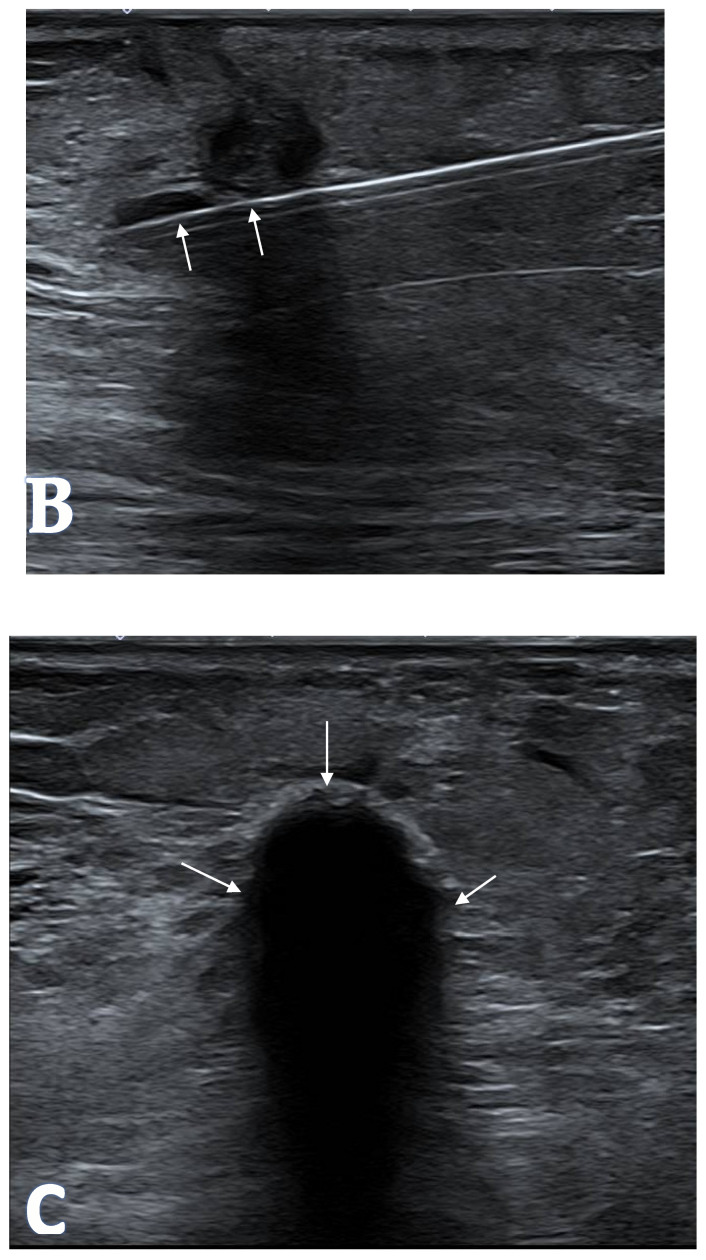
Ultrasound characteristics of a patient’s deltoid parathyroid autoimplant (male, 68 years old, on hemodialysis for 10 years) before and after cryotherapy (CA). (A) A 3.5 × 2.5 cm hypoechoic parathyroid autoimplant in the deltoid muscle before cryotherapy: normal parathyroid autoimplant on the left (white arrow) and hyperplastic parathyroid autoimplant on the right (bolded white arrow). (B) Cryo probe (white arrow) was inserted in the hypoechoic parathyroid autoimplant. (C) Hypoechoic “ice ball” (white arrow) was formed inside the parathyroid gland.
